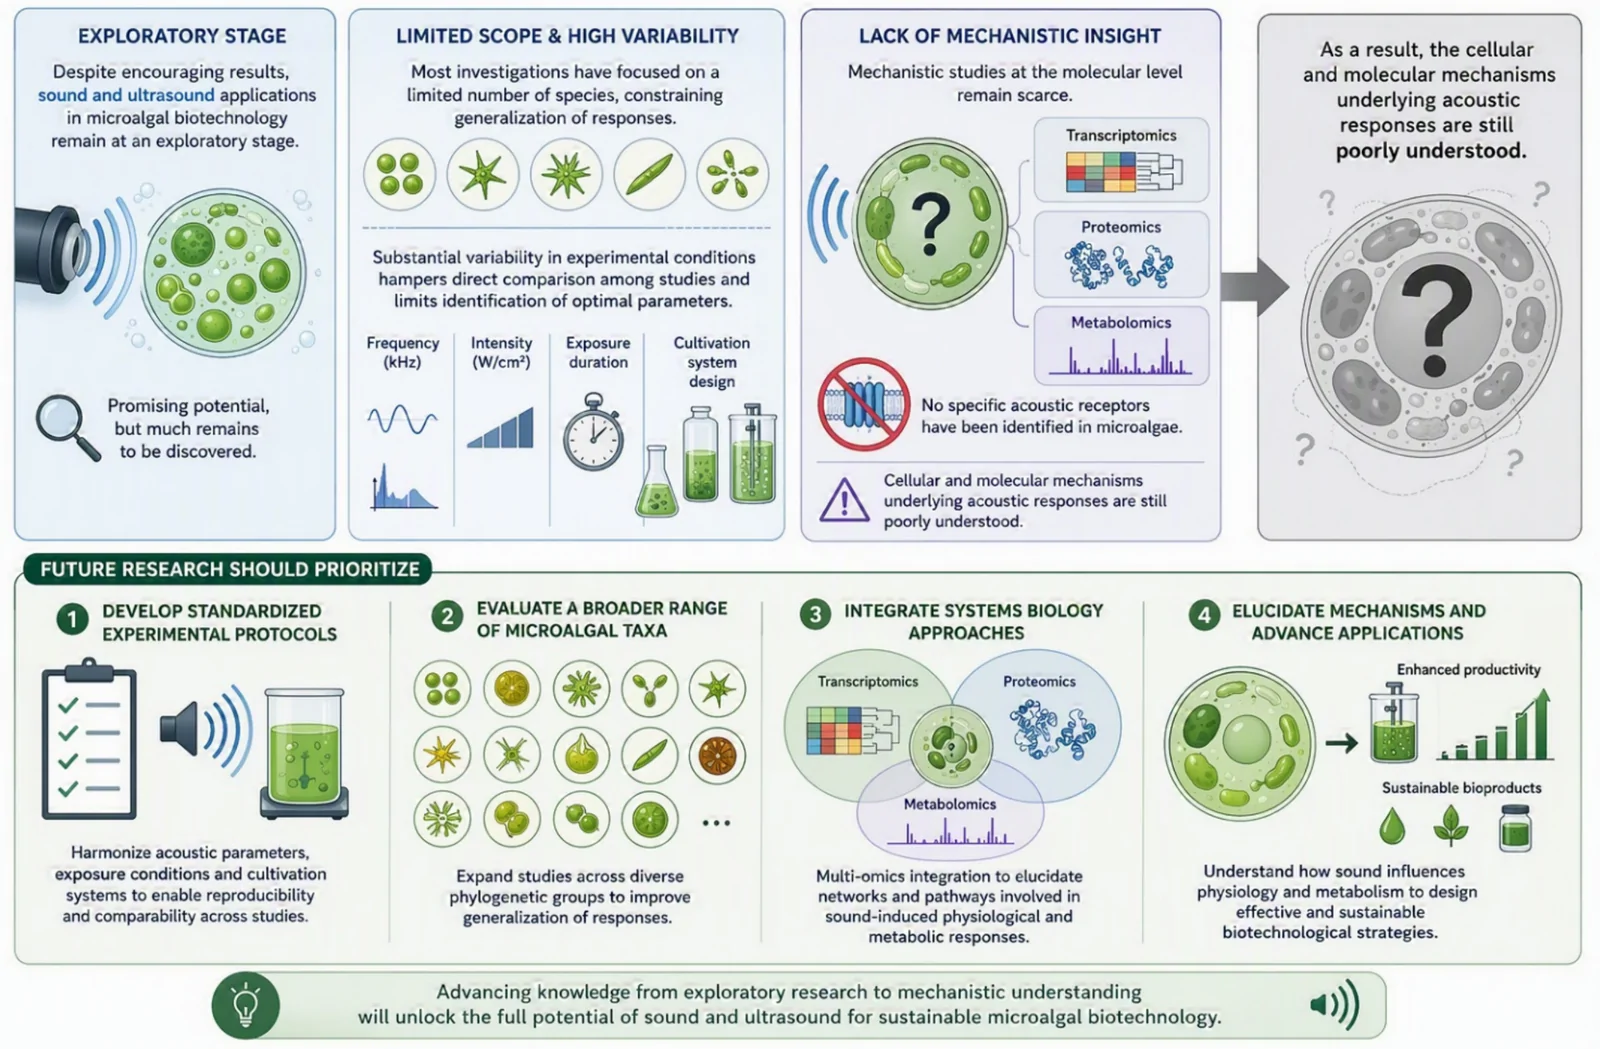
Current limitations and future research priorities for the application of sound and ultrasound in microalgal biotechnology.
